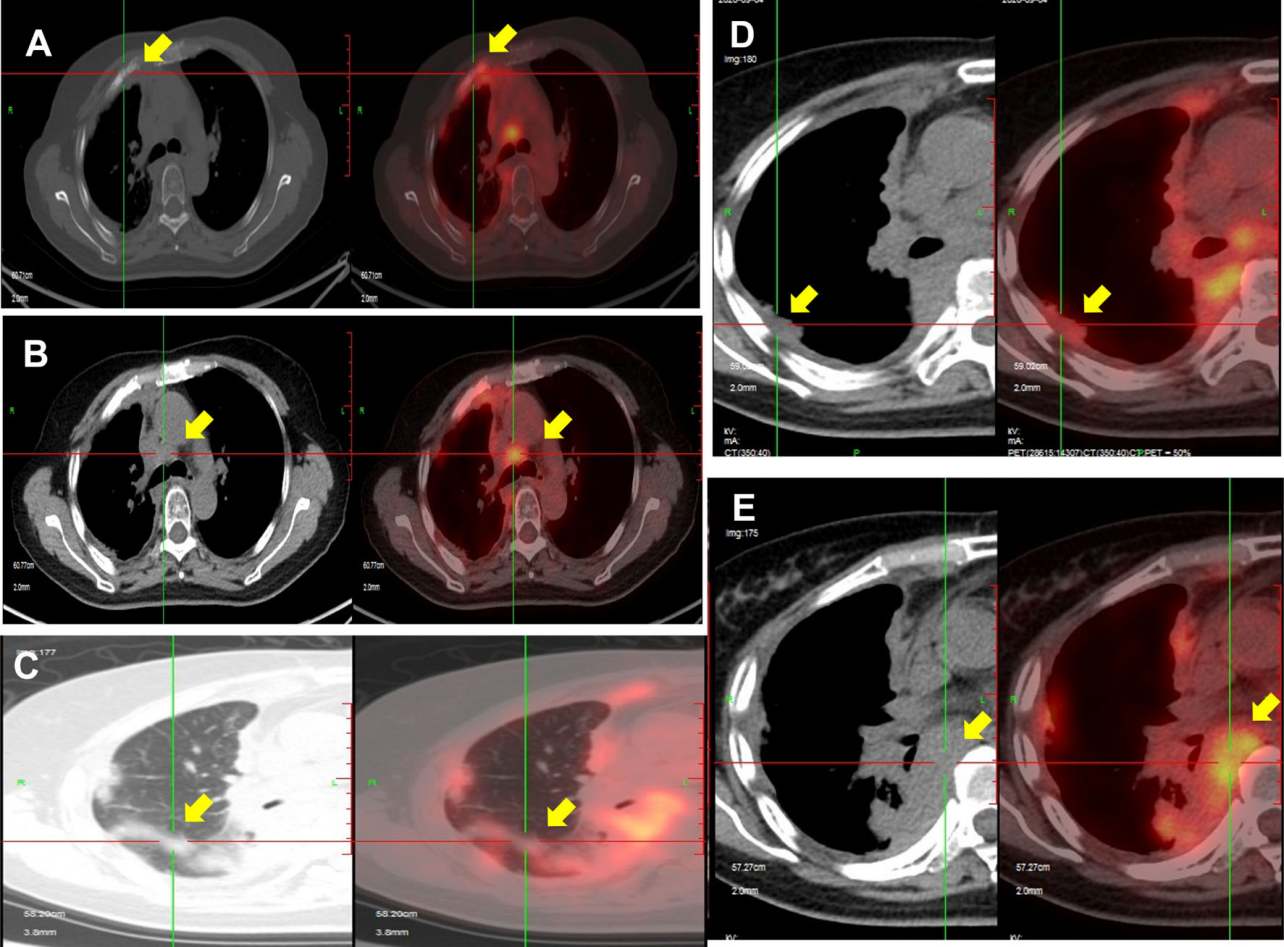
PET/CT scan in September 2020 showing disease progression after radiotherapy. Bone metastases (A), lymph nodes in the mediastinum and diaphragm (B, E), lung metastases (C), and pleural metastases (D) show progression compared with scans in April 2020.
